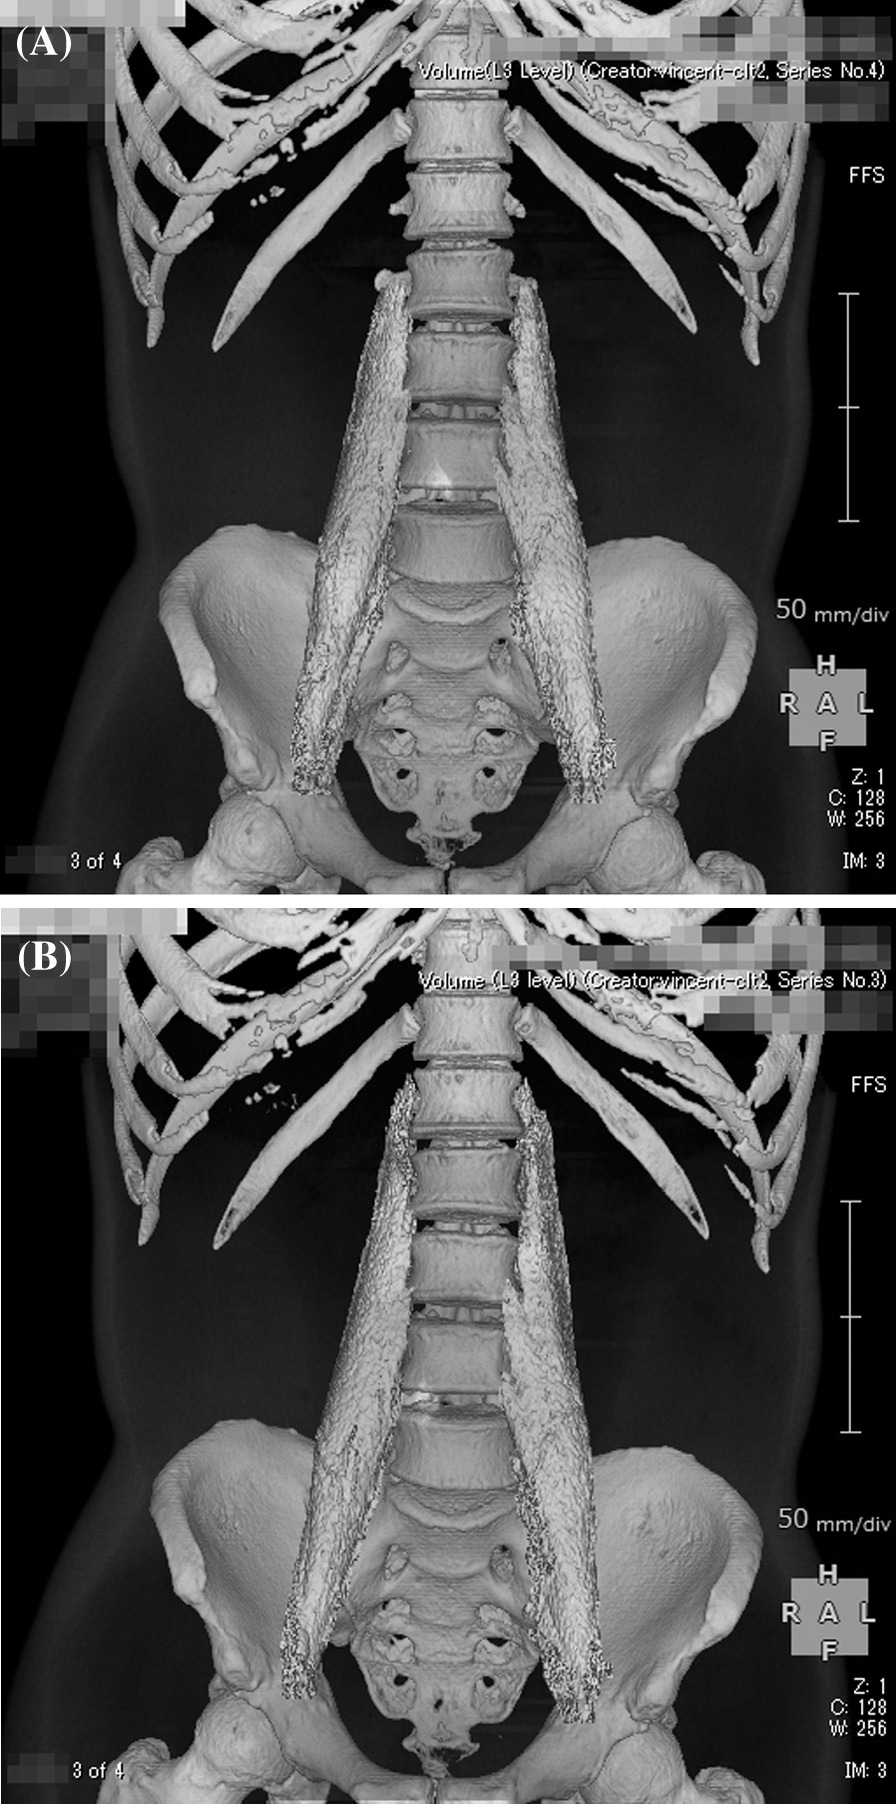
Reconstructed computed tomography images of the psoas major muscle taken at: A day T + 41 (muscle mass 276 cm^3^), B day T + 116 (muscle mass 316 cm^3^)
